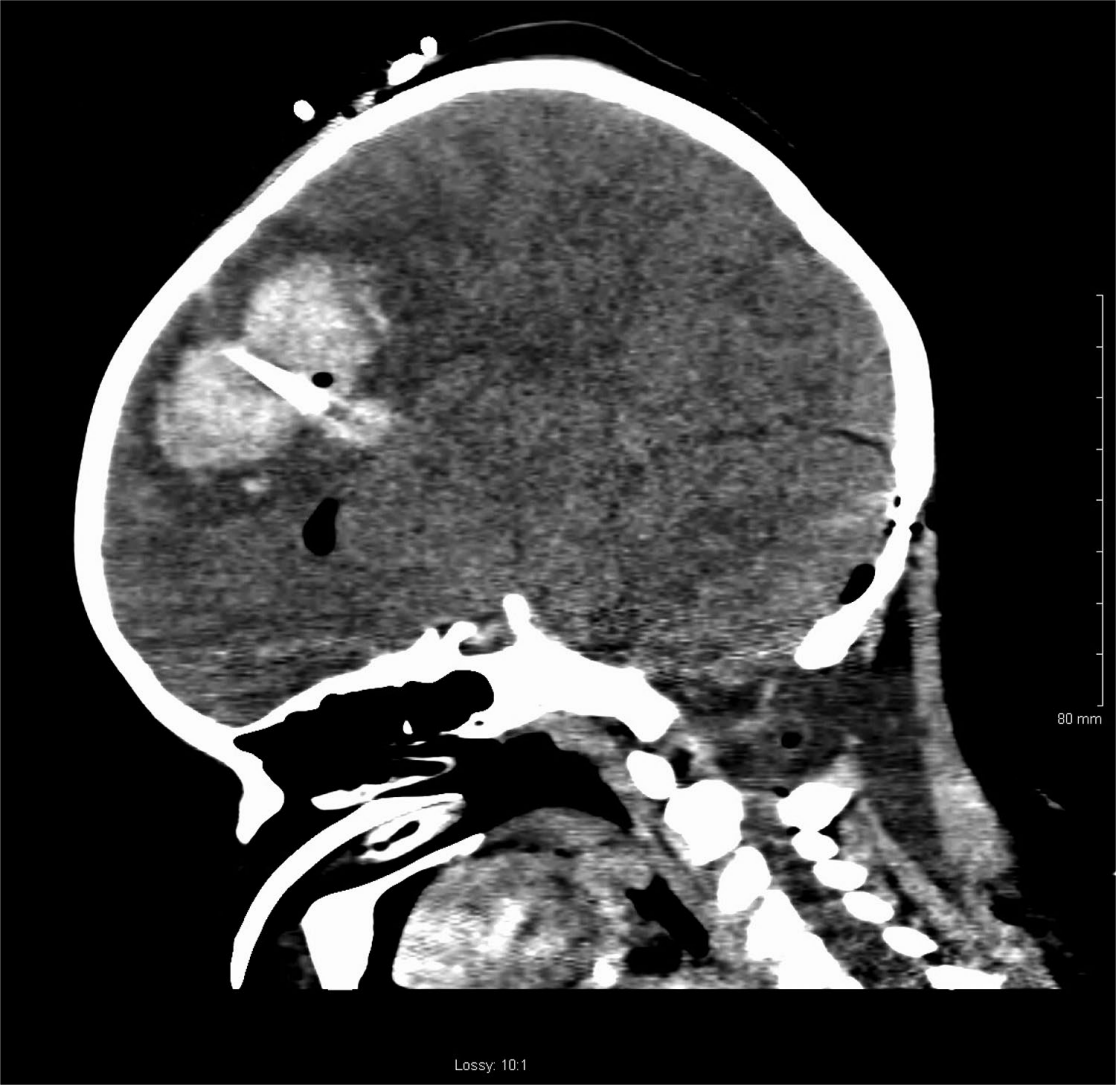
Postoperative CT brain (sagittal view) of spontaneous intraparenchymal haemorrhage along right frontal EVD tract
